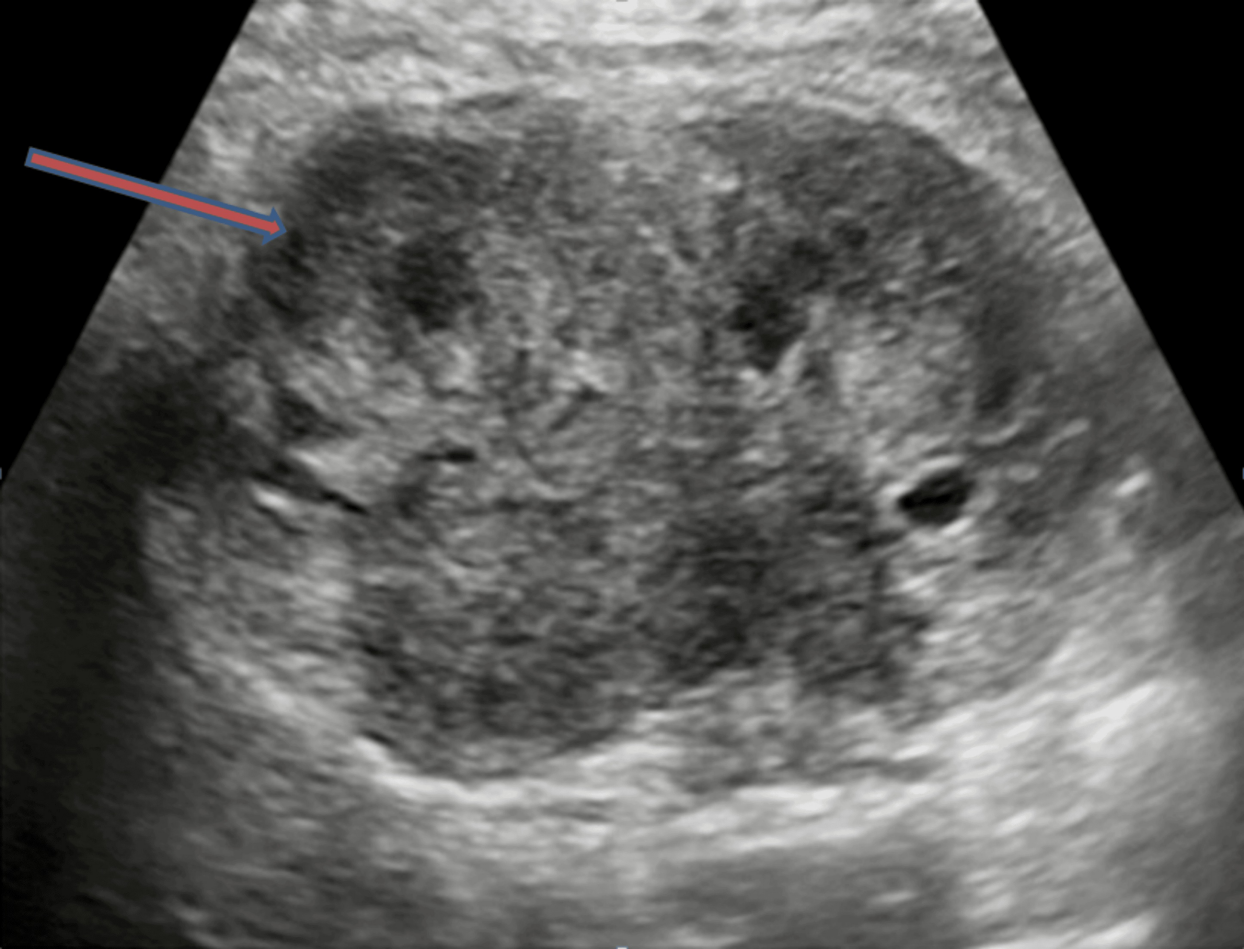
The ultrasound image demonstrates a heterogeneous hypoechoic lesion predominantly located in the hilar and perihilar regions of the kidney. The mixed echogenicity within the lesion indicates varying tissue densities. The hypoechoic nature suggests less dense tissue than the surrounding renal parenchyma. The central location potentially affects the renal pelvis and major blood vessels, indicative of a malignant tumor.
